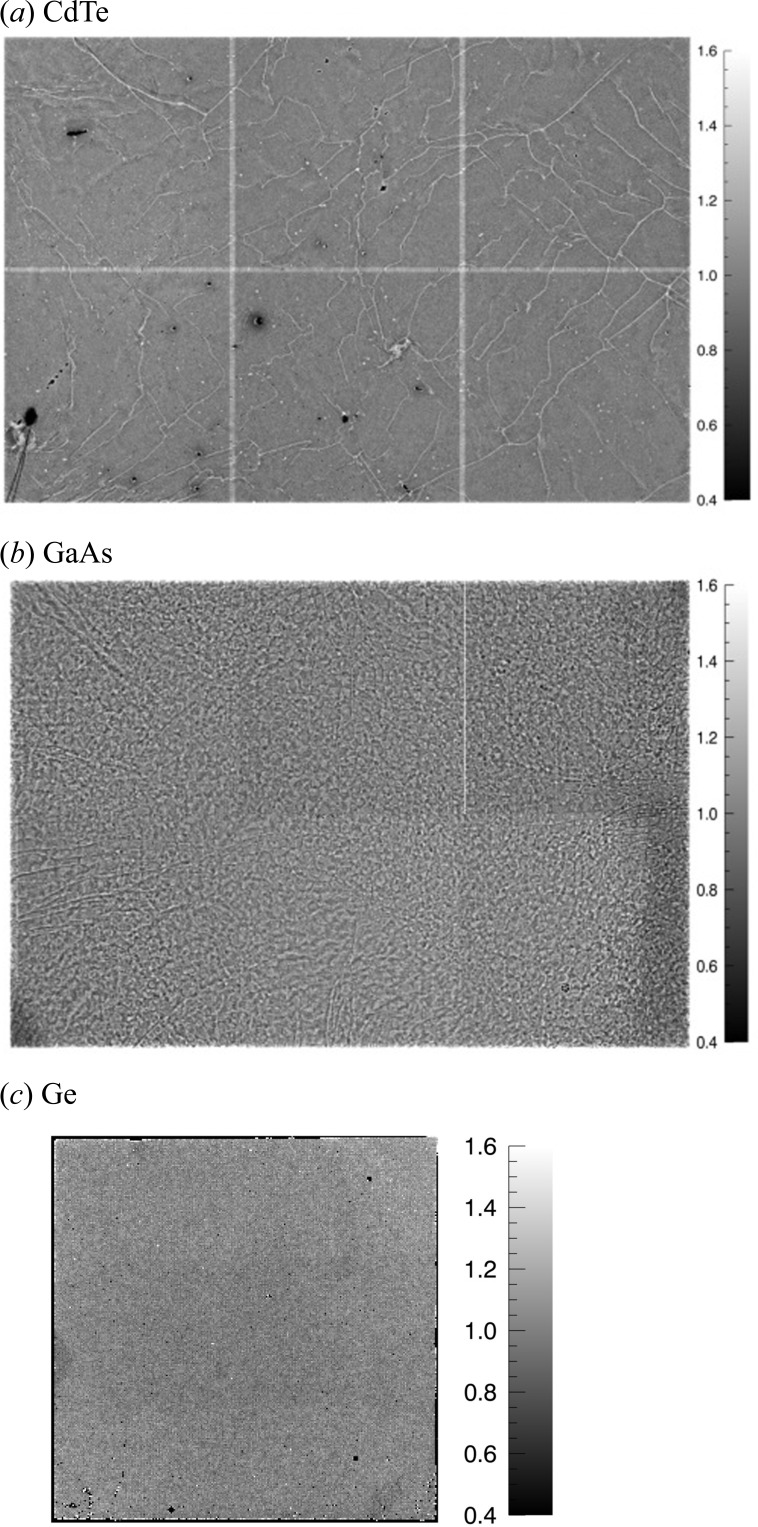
Flat-field images of high-*Z* sensors bump-bonded to 3 × 2 Medipix3 readout chips, taken using an X-ray source at 40 keV with an Mo target for sensors of (*a*) CdTe and (*b*) GaAs (Pennicard, Smoljanin *et al.*, 2014 ▶). The scales indicate the relative gain. (*c*) An image of a Ge sensor bump-bonded to a Medipix3 readout chip, taken using an X-ray source at 40 keV with an Ag target (Pennicard, Struth *et al.*, 2014 ▶). (Copyright SISSA Medialab Srl. Reproduced by permission of IOP Publishing. All rights reserved.)
